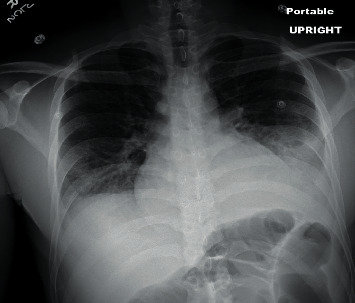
Chest X-ray revealing bilateral pleural effusions right middle lobe consolidation.
